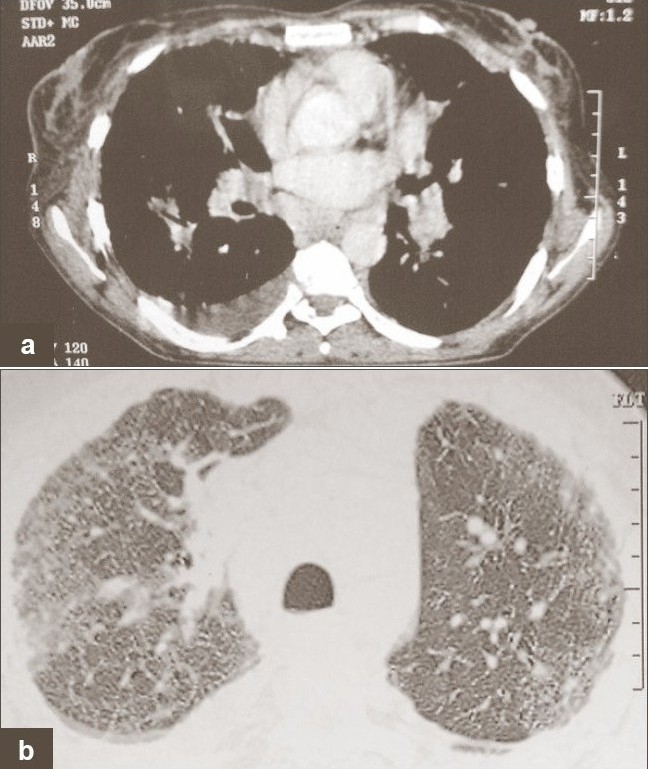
(a, b) Computed tomography revealed bilateral hilar lymphadenopathy with parenchymal opacity and left-sided pleural effusion
